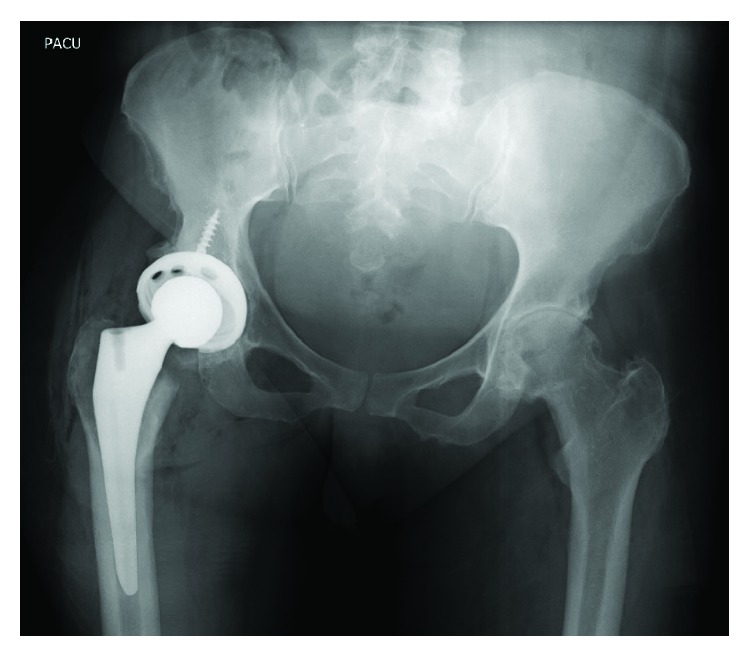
Postoperative AP pelvis radiograph after the right total hip arthroplasty.
